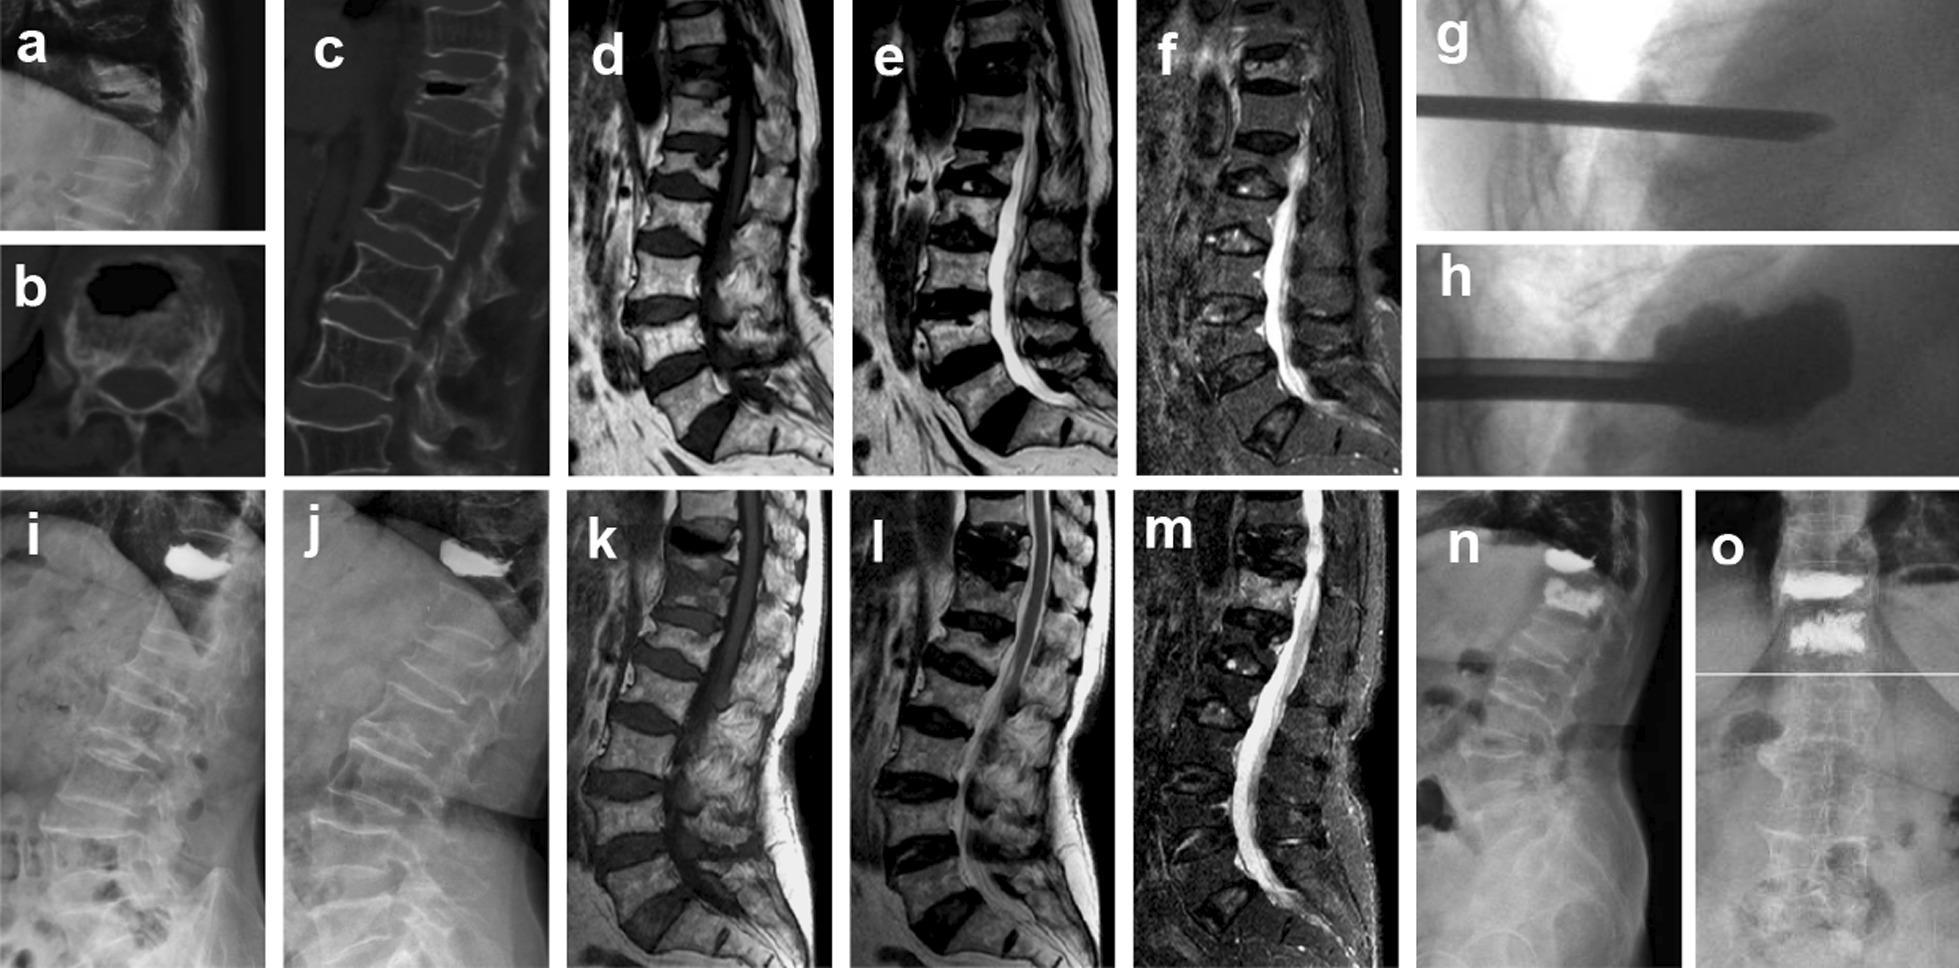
Treatment of Kümmell’s disease via percutaneous vertebroplasty (PVP). A female aged 73 years complained of low back pain and discomfort for 6 months. Preoperative lateral X-ray suggested a reduction in the height of the T11 vertebral body a Preoperative computed tomography (CT) revealed dehiscence of the anterior middle region of vertebra T11 and the formation of a vacuum fissure within the vertebra b, c Preoperative magnetic resonance imaging confirmed that the T11 vertebral body was a fresh fracture. The vacuum fissure in the vertebral body also evidenced a fluid signal d–f The patient underwent PVP. Intraoperative bone cement injection was monitored using X-ray fluoroscopy g Intraoperative lateral X-ray after PPP h An X-ray taken on the first day after surgery revealed that the bone cement was evenly distributed in the vertebral body i An X-ray taken on the 3 years after surgery revealed that the bone cement forward migration and lateral fusion with the adjacent vertebral body, the height of the T12 vertebral body was a reduction j Preoperative magnetic resonance imaging confirmed that the T12 vertebral body was a fresh fracture k–m A postoperative lateral and anteroposterior X-ray after PVP n, o
